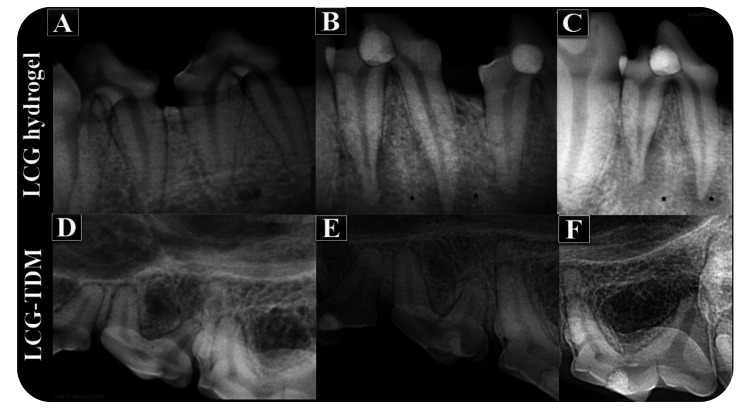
Periapical radiographic examination of teeth treated with LCG and LCG-TDM, respectively. (A and D) Preoperative periapical radiographs showing sound teeth and normal periapical tissues. (B and C) Postoperative periapical radiographs showing periapical pathology with root resorption after 8 weeks of treatment with LCG before extraction. (E and F) Postoperative periapical radiographs showing no sign of periapical pathology after 8 weeks of treatment with LCG-TDM before extraction
